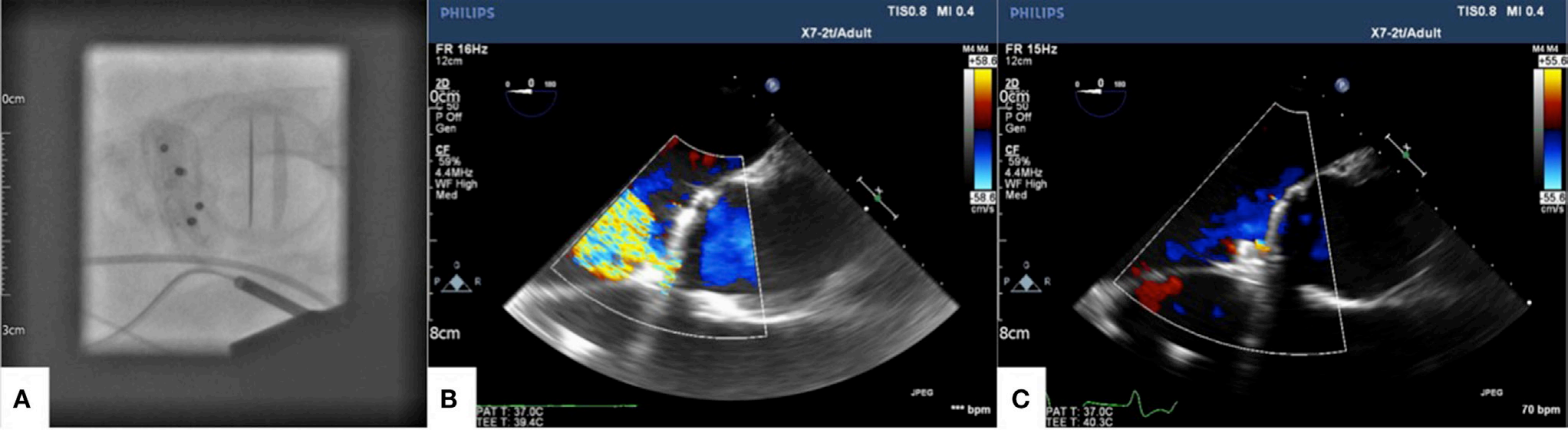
AVP3 14/5 devices shown in final position in short axis on fluoroscopy (A). Color Doppler showing baseline paravalvular leak (B) and final result after device closure (C) on intraoperative transoesophageal echocardiography.
